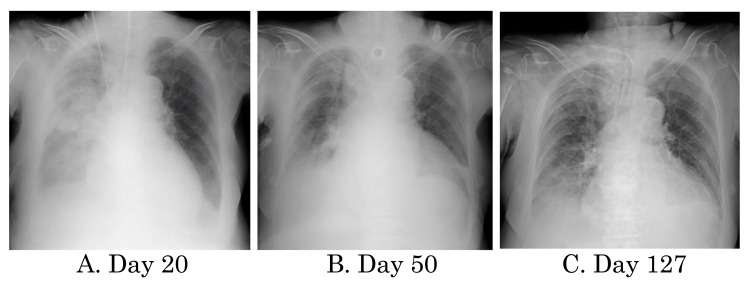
Chest X-rays on hospital admission day 20 (A), day 50 (B), and day 127 (C).
